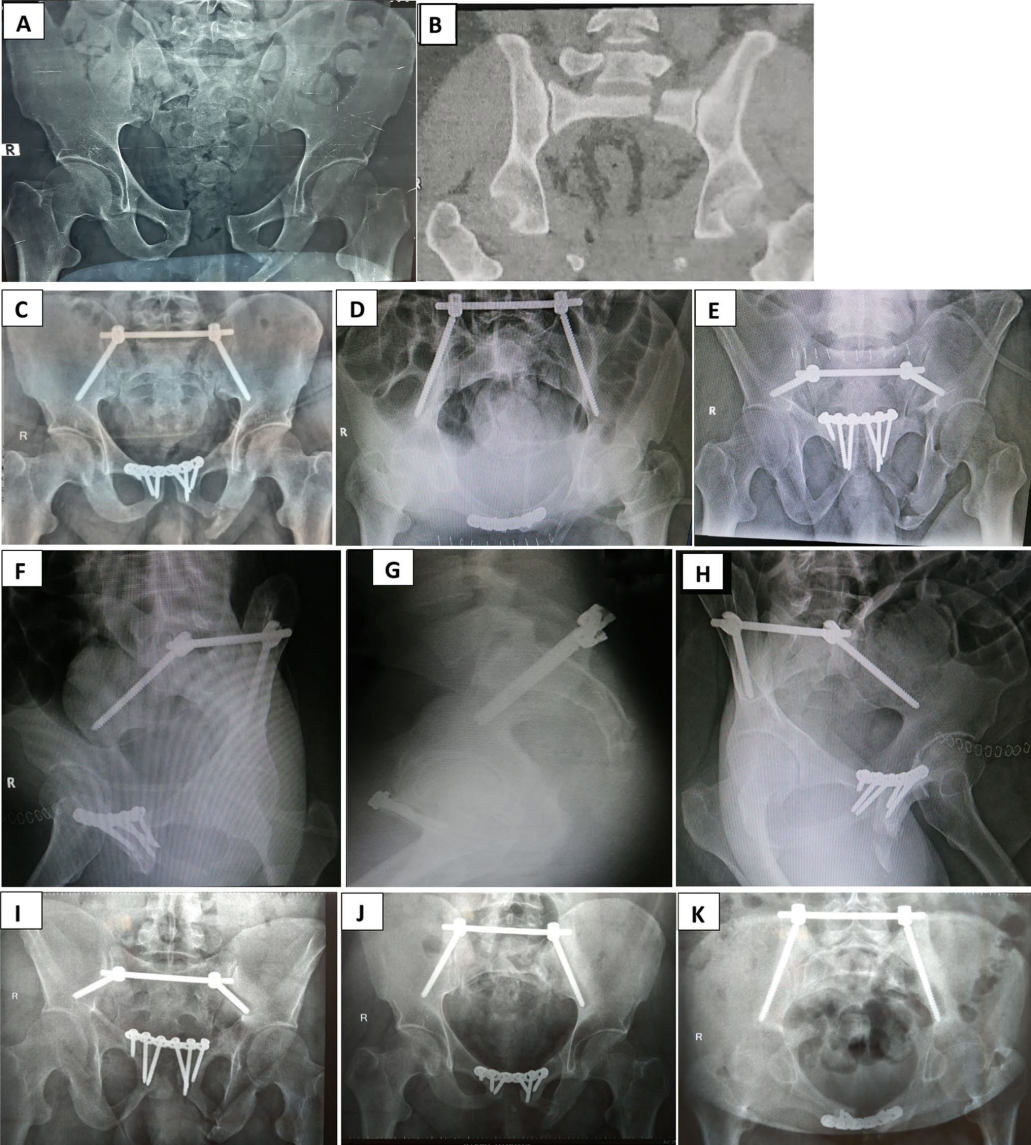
A Preoperative radiograph showing left fracture sacrum Denis type 2 in 40 years old male. B–H Postoperative radiographic views showing iliac screws accurate trajectory. I–K Final follow-up radiographs
